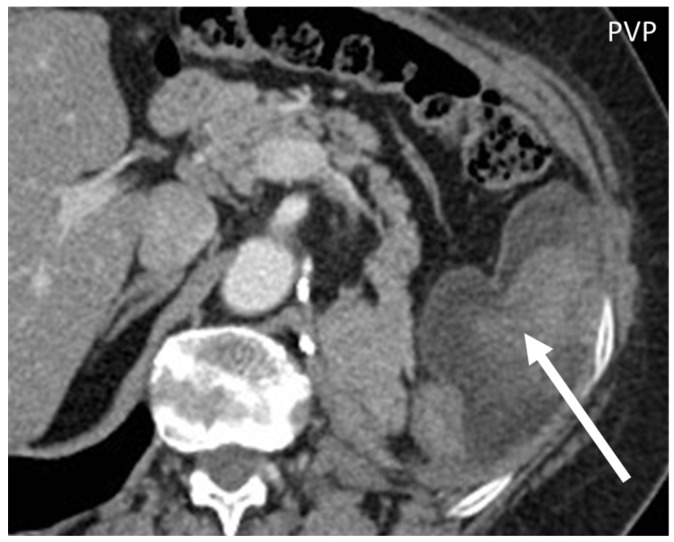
Axial, portal venous phase CT shows the appearance of a well-differentiated liposarcoma (arrow) of the left transverse muscle in a 63-year-old woman, presenting as a lobulated mass with fat peripheral attenuation and slight enhancement.
